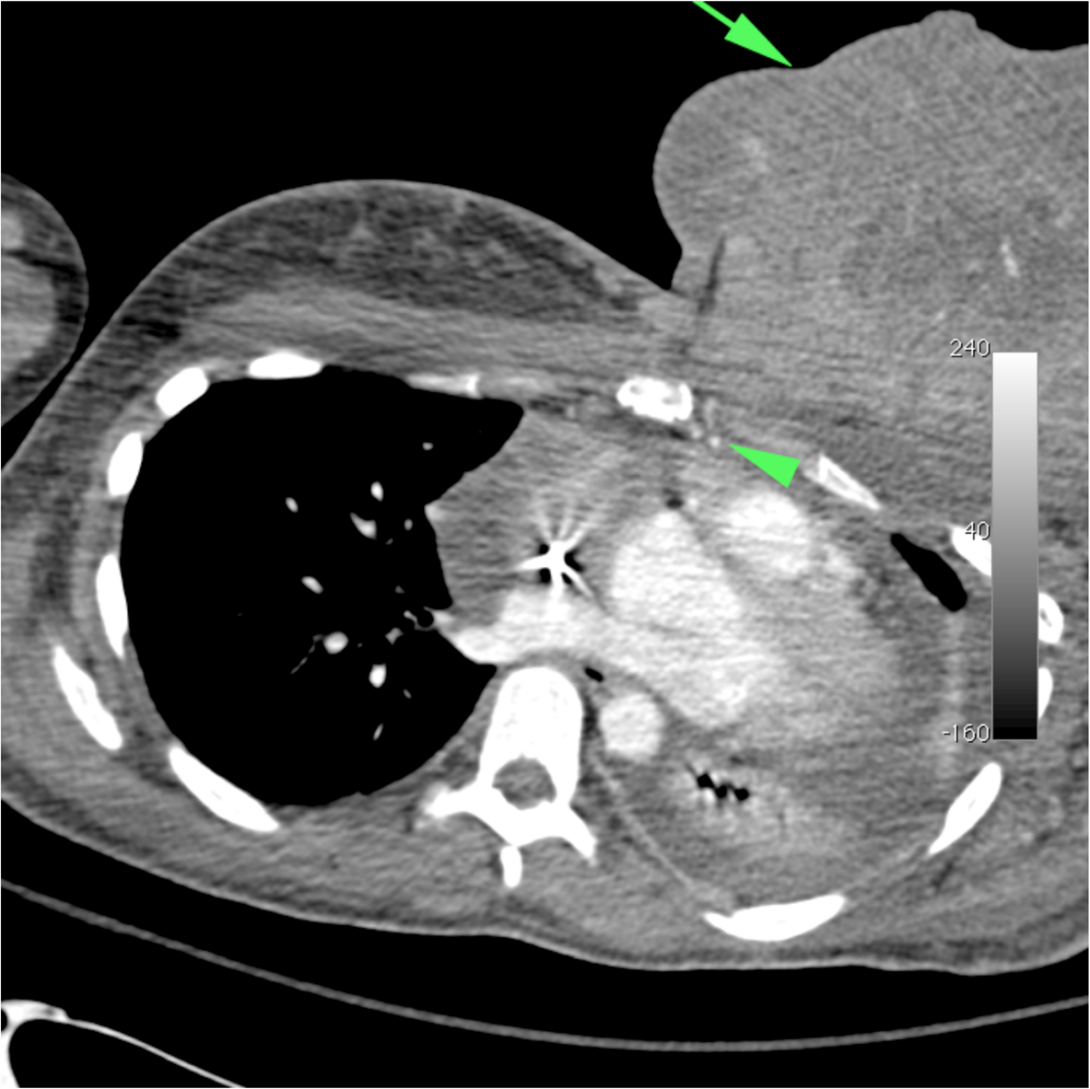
Axial contrast-enhanced computed tomography image of the thorax demonstrates a 14cm enhancing breast mass (arrow). Branch vessels of the left internal mammary artery (arrowhead) passing anteriorly into the mass were noted.
